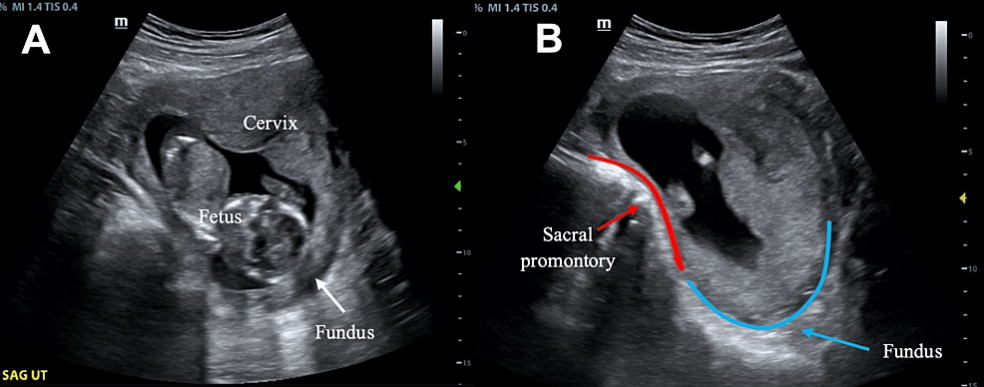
Point-of-care transabdominal ultrasound images of incarcerated gravid uterus in the sagittal plane. A) Sagittal image demonstrates the superiorly displaced cervix and lower uterine segment above the uterine fundus. B) Parasagittal image shows the uterus is fixed in retroversion with the fundus (marked by the blue line) trapped below the sacral promontory (marked by the red line) seen while fanning the ultrasound transducer through the sagittal and parasagittal planes.
